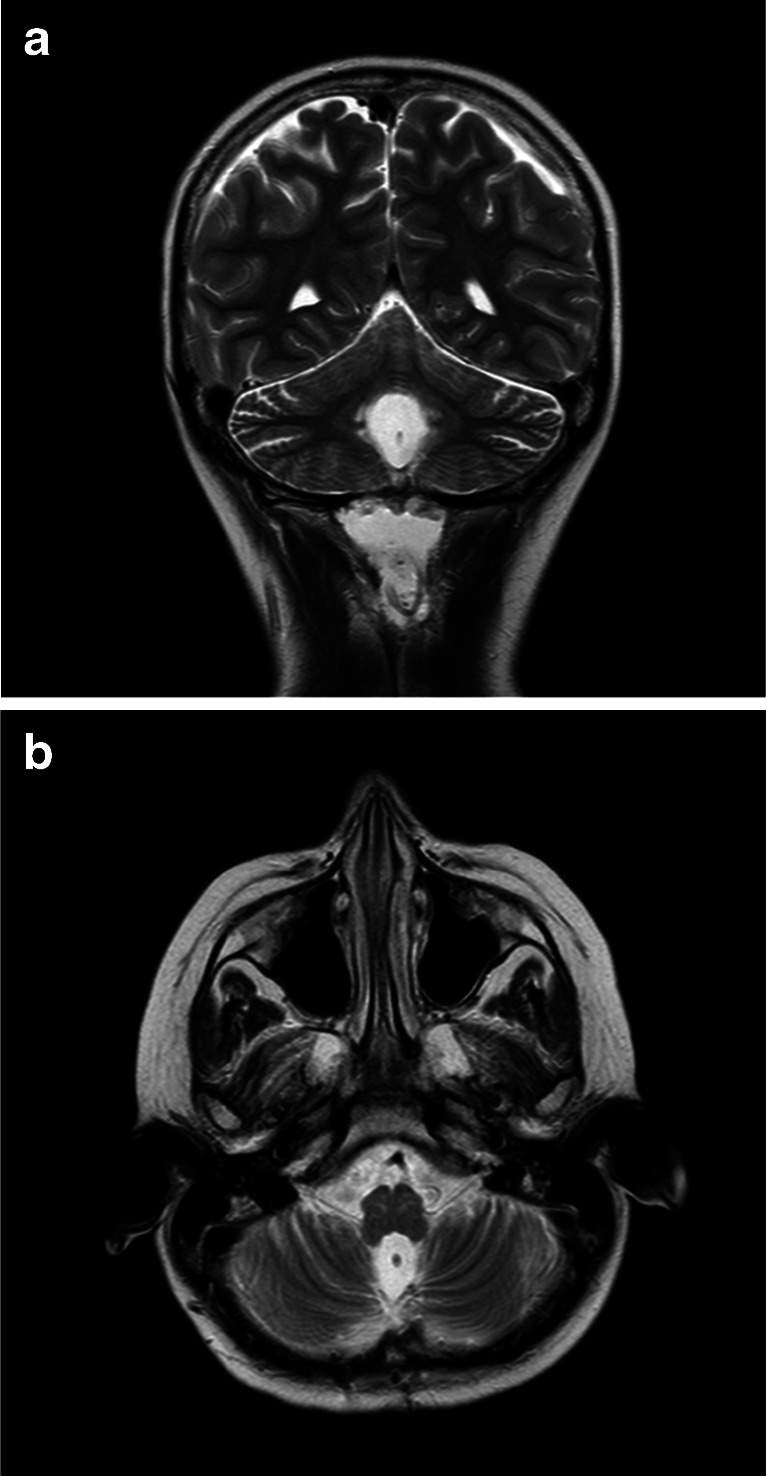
Postoperative T2-weighted MRI scans demonstrating the ventricular catheter in position in the fourth ventricle. The catheter is marked by an *arrow* in coronal (a) and axial (b) views
